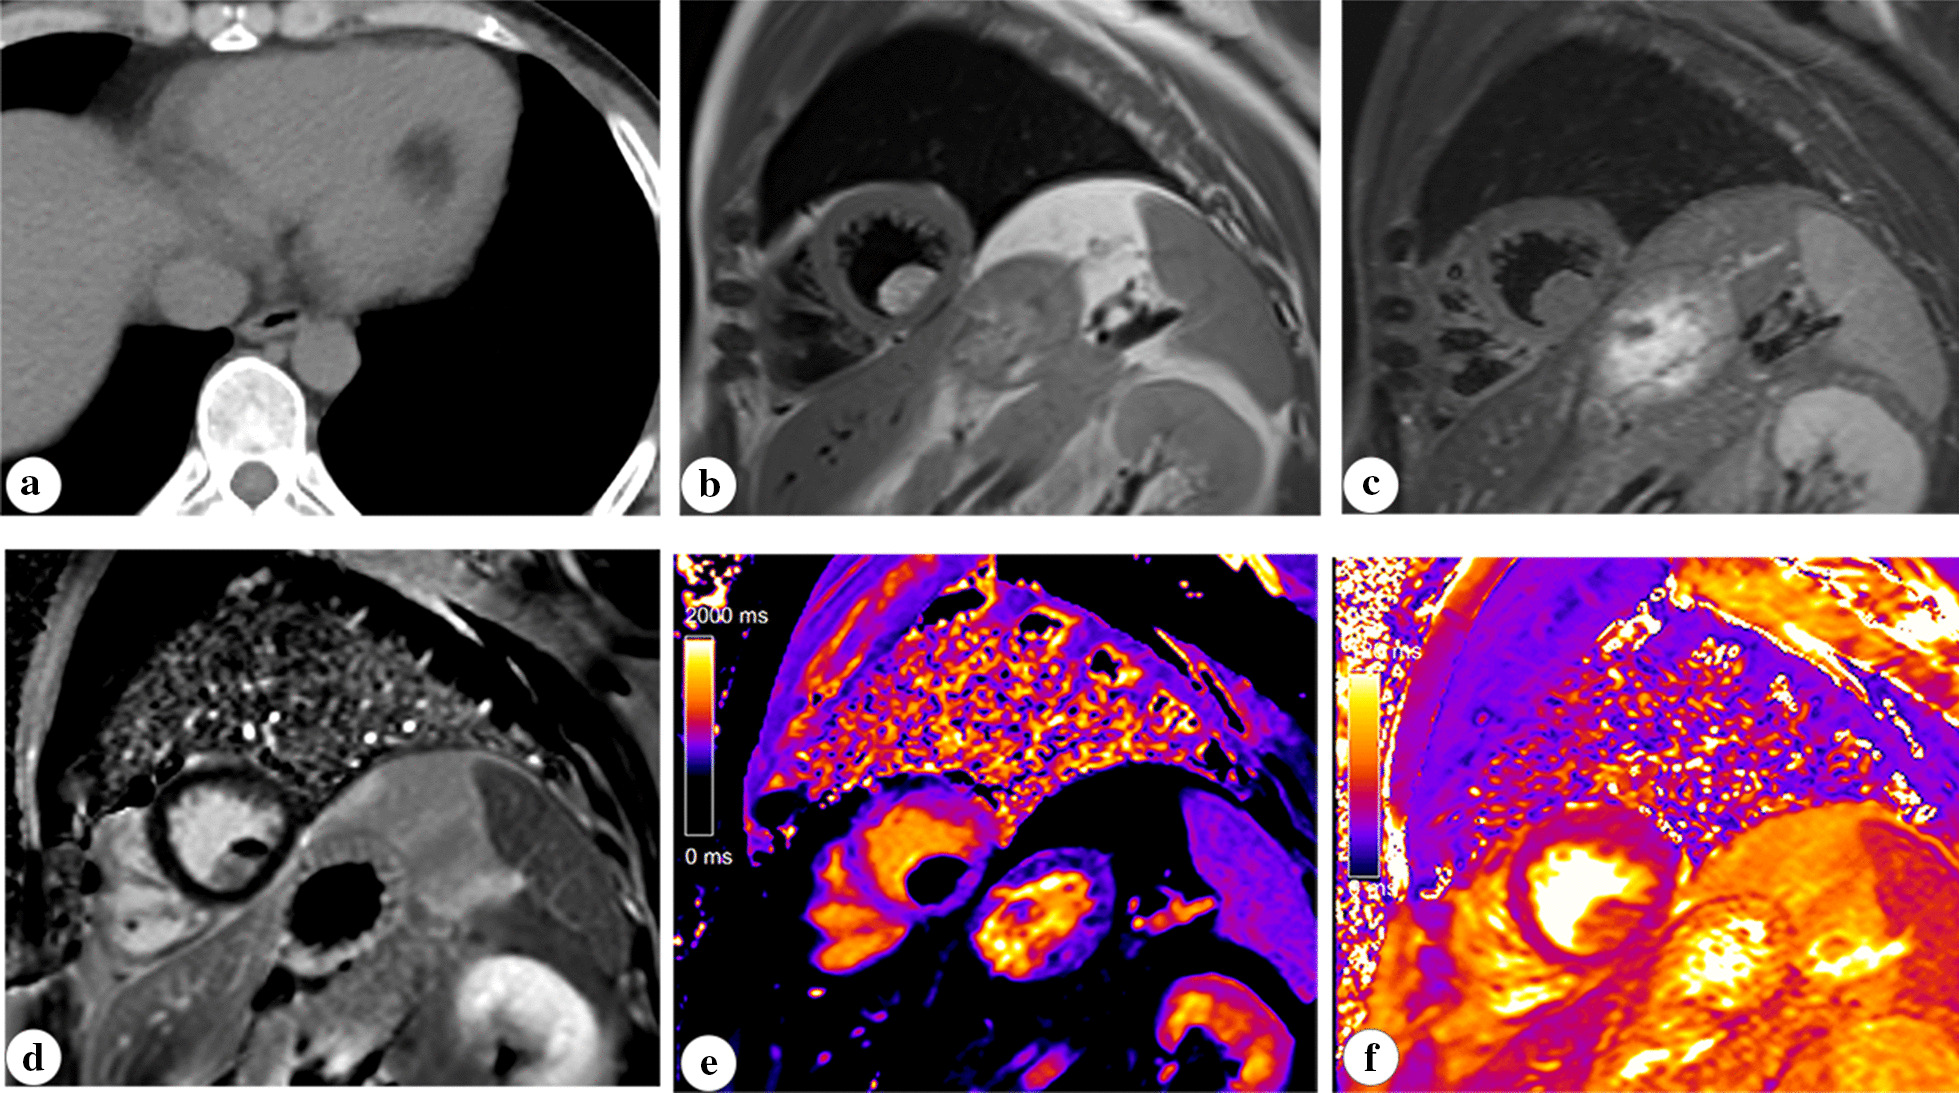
CT and cardiac MR images of a sessile ventricular lipoma in an asymptomatic male patient. The attenuation value in CT (a, − 45 HU) and characteristic findings in MRI (b, c, d) of the mass confirmed the nature of fat tissue. Besides, the ventricular mass showed same T1 (244 ms) and T2 values (61 ms) as subcutaneous fat in tissue mapping sequences (e, f)
